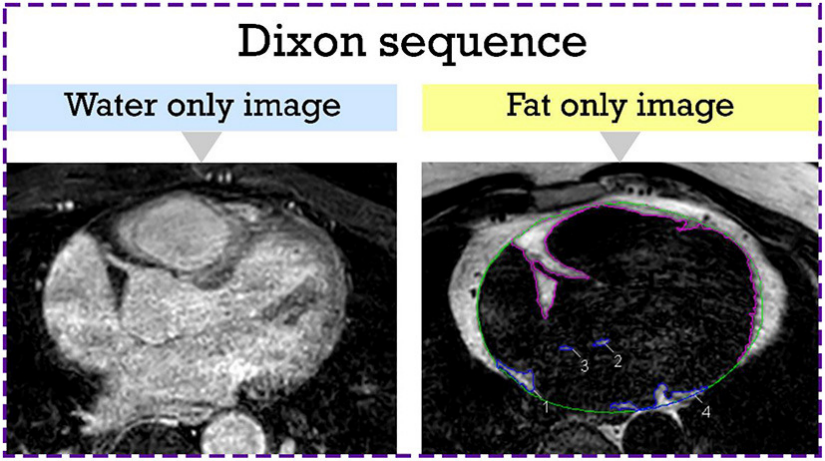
Epicardial adipose tissue segmentation from the axial Dixon MRI sequence. Hyperintense regions around the LA which represent LA EAT are manually contoured in blue. The rest of the EAT is contoured in purple. The pericardium is outlined in green. EAT, epicardial adipose tissue; LA, left atrial.
